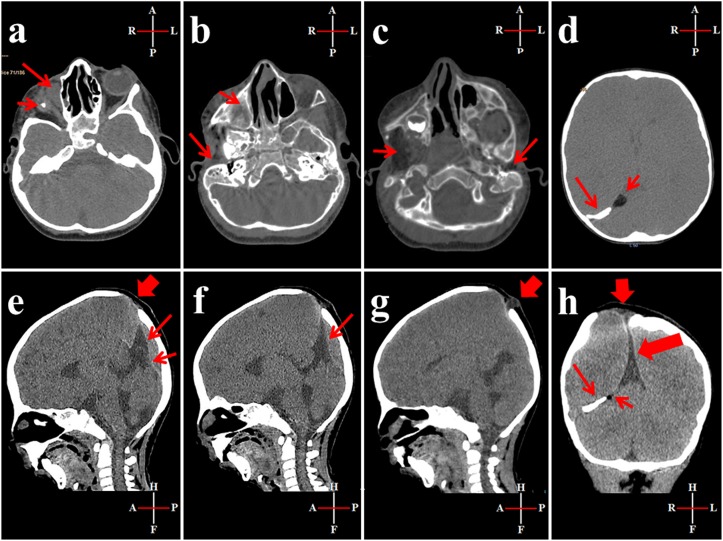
CT scan of the head a.
